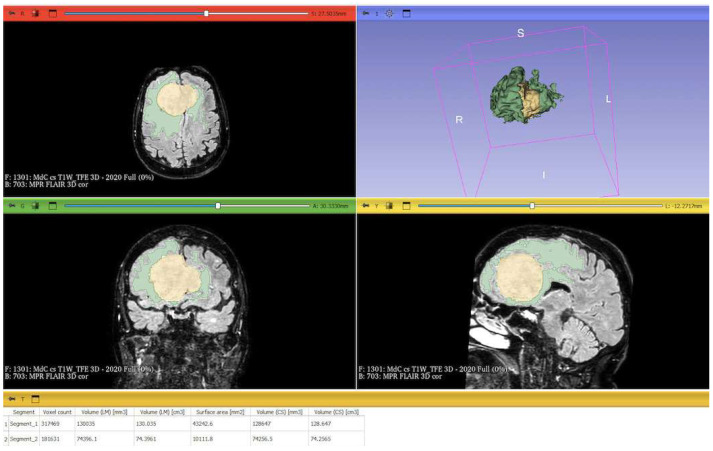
Screenshot of 3D Slicer showing the completed segmentation of the meningioma and PE, with 3D reconstruction and statistical quantification: the segmented PE is shown in green; the segmented meningioma is shown in yellow; and at the bottom, the statistical quantification of the segment volumes is displayed. The MRIs include a T1-weighted sequence and a FLAIR sequences merged at 50%. PE: peritumoral edema; MRI: magnetic resonance image; FLARI: fluid-attenuated inversion recovery.
